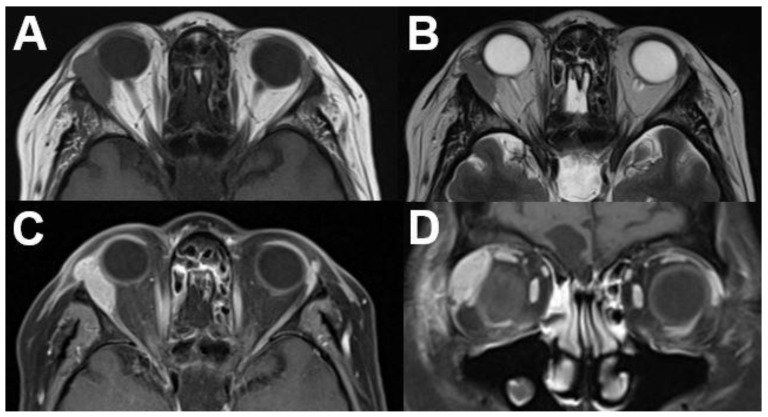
Mucosa-associated lymphoid tissue (MALT) lymphoma.* MRI of a 54-year-old female with a right-sided primary orbital MALT lymphoma of the lacrimal gland. T1-weighted (A) and T2-weighted (B) images show a well-defined lobulated tumor with homogenous contrast enhancement on axial (C) and coronal view (D). Infiltration of adjacent structures cannot be identified.
